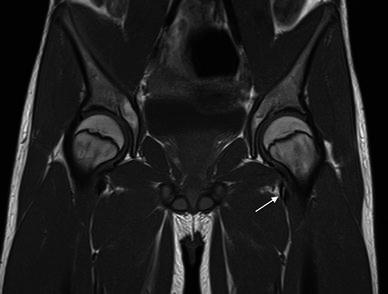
Coronal T1-weighted image, with *arrow* showing the bifid iliopsoas tendon at level of the femoral neck
